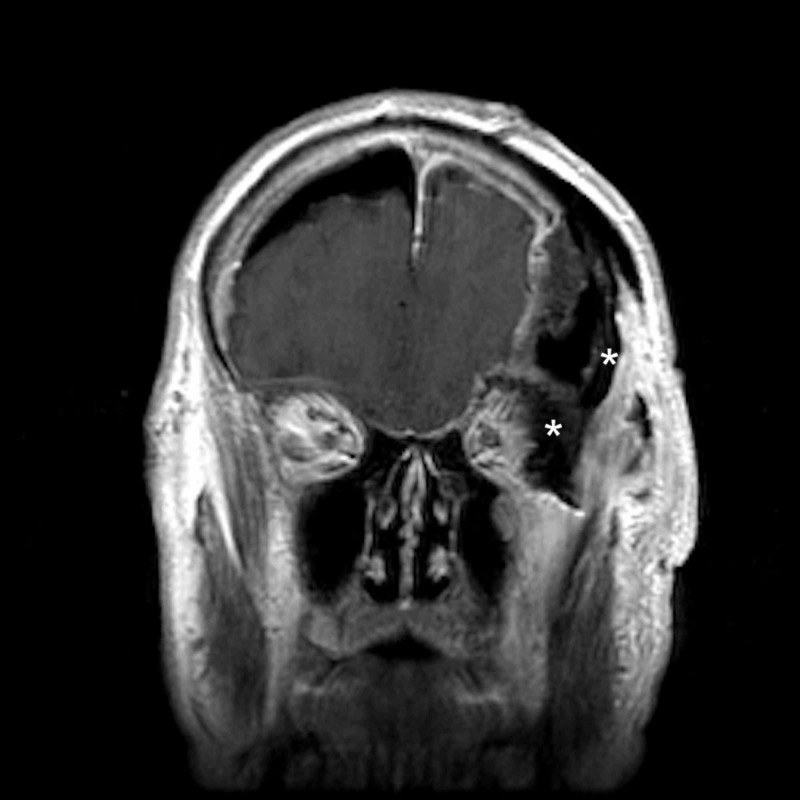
Postoperative coronal MRI demonstrating resection of the intraorbital and intramuscular tumor component * = Areas of prior tumor invasion of the lateral orbit and temporalis muscle MRI: magnetic resonance imaging
